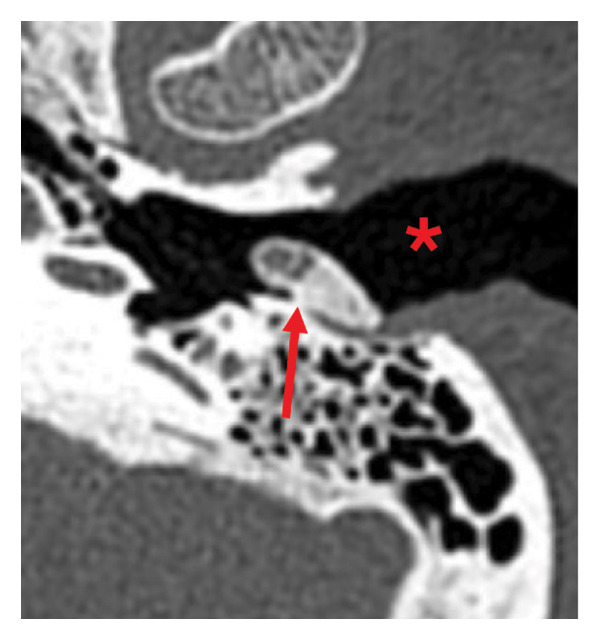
Axial CT of the left temporal bone from 6 years prior to patient presentation, depicting a pedunculated bony lesion within left EAC consistent with EAC osteoma. The red arrow indicates the stalk of the osteoma. The asterisk (∗) denotes the lateral and cartilaginous portion of the EAC.
